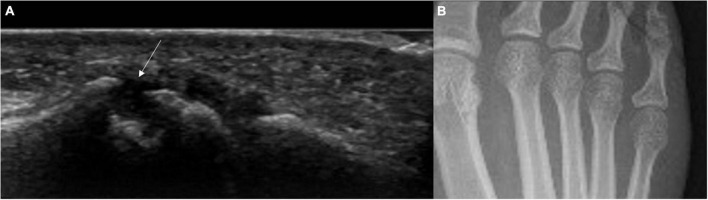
US bone erosion (not detected by x-rays) in the 5th MTP joint in an individual with high titre ACPA and non-specific MSK symptoms. (A) Longitudinal US scan of the lateral aspect of the 5th MTP joint. The white arrow indicates the presence of bone erosion. (B) Correspondent feet x-rays showing no bone erosions in the 5th metatarsophalangeal joint. This patient presented with non-specific musculoskeletal symptoms and high titre anti-CCP antibodies.
